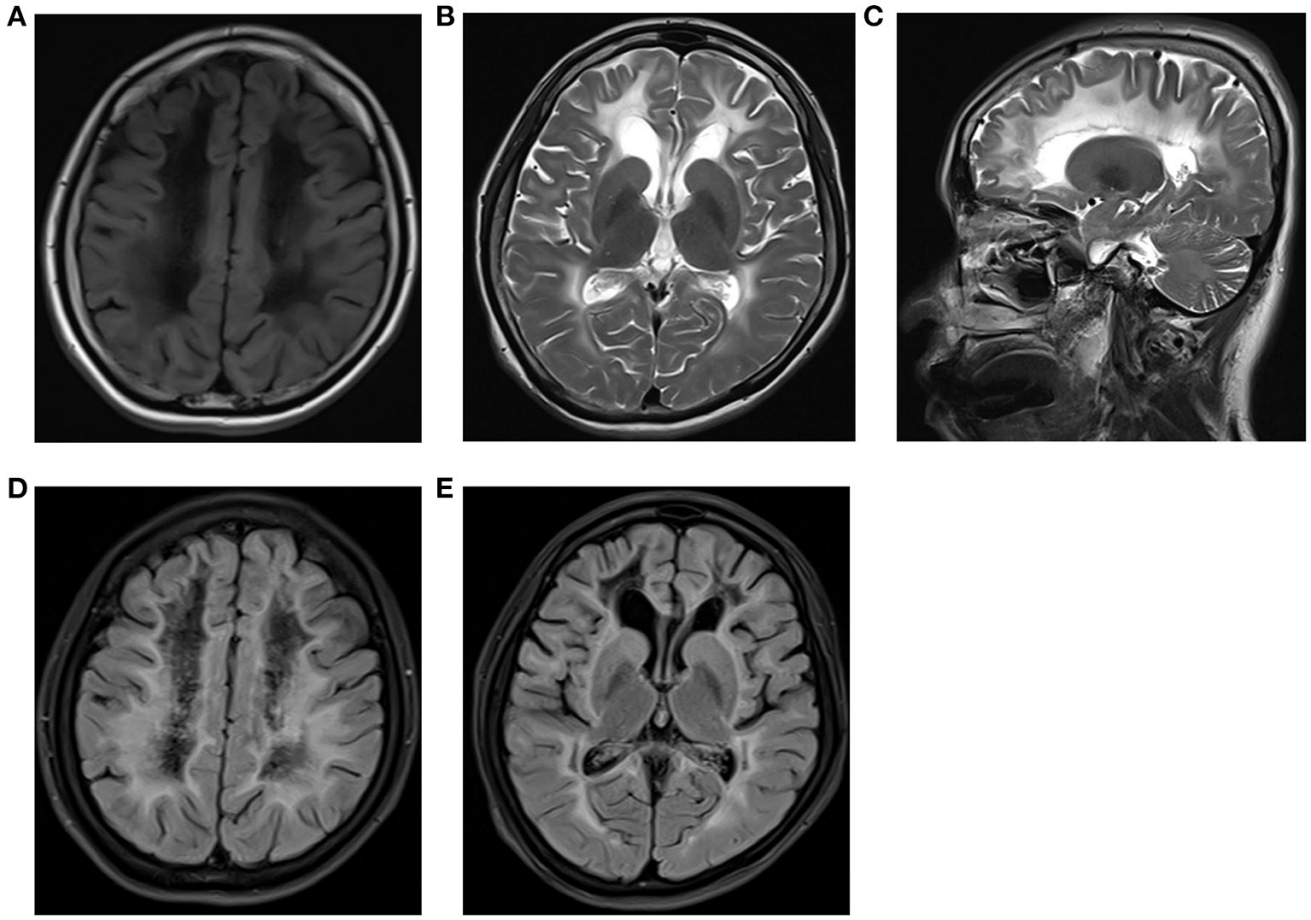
Magnetic resonance imaging showing extensive and symmetrical changes in bilateral cerebral hemisphere demyelination with glial hyperplasia involving the corpus callosum. Obvious brain atrophy was also observed. (A) Axial T1-weighted. (B) T2- weighted. (C) Coronal T2-weighted. (D, E) T2Flair.
